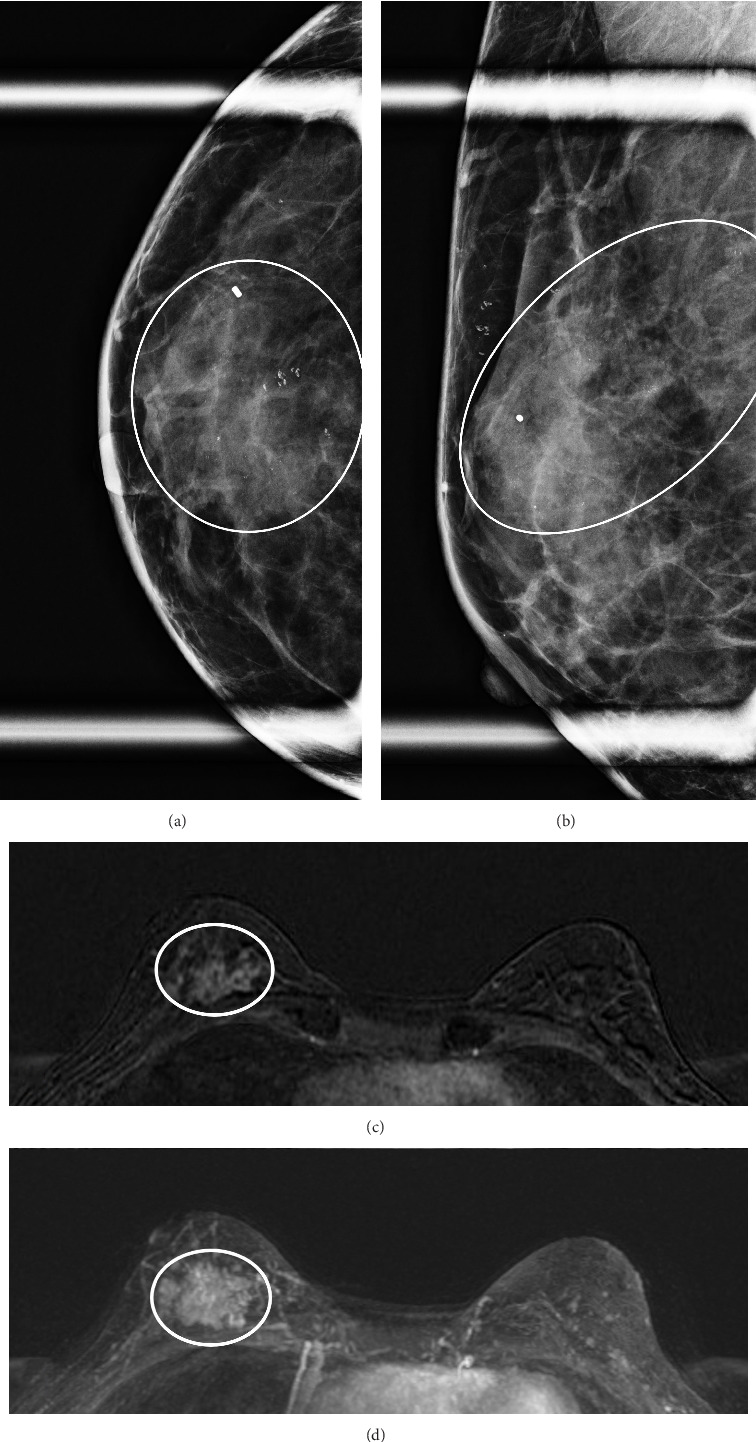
(a and b) Right breast magnification CC and ML views show segmentally distributed coarse heterogeneous calcifications. (c and d) Breast MRI T1 subtraction and maximum intensity projection axial sequences show regionally distributed clumped nonmass enhancement in the right breast.
